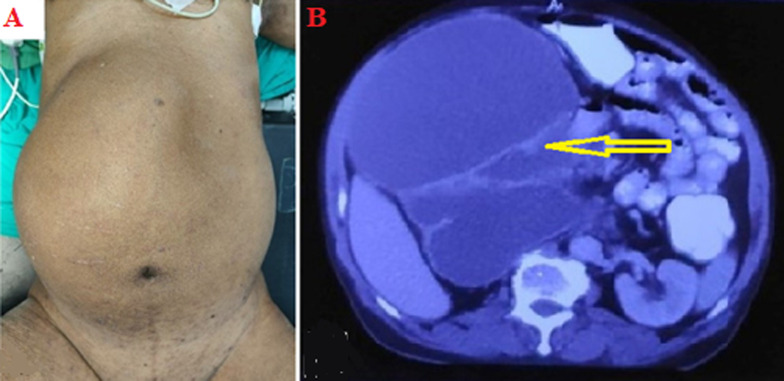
A) preoperative photo showing a distended abdomen; B) computerised tomography (CT) scan showing right giant hydronephrosis (arrow)
